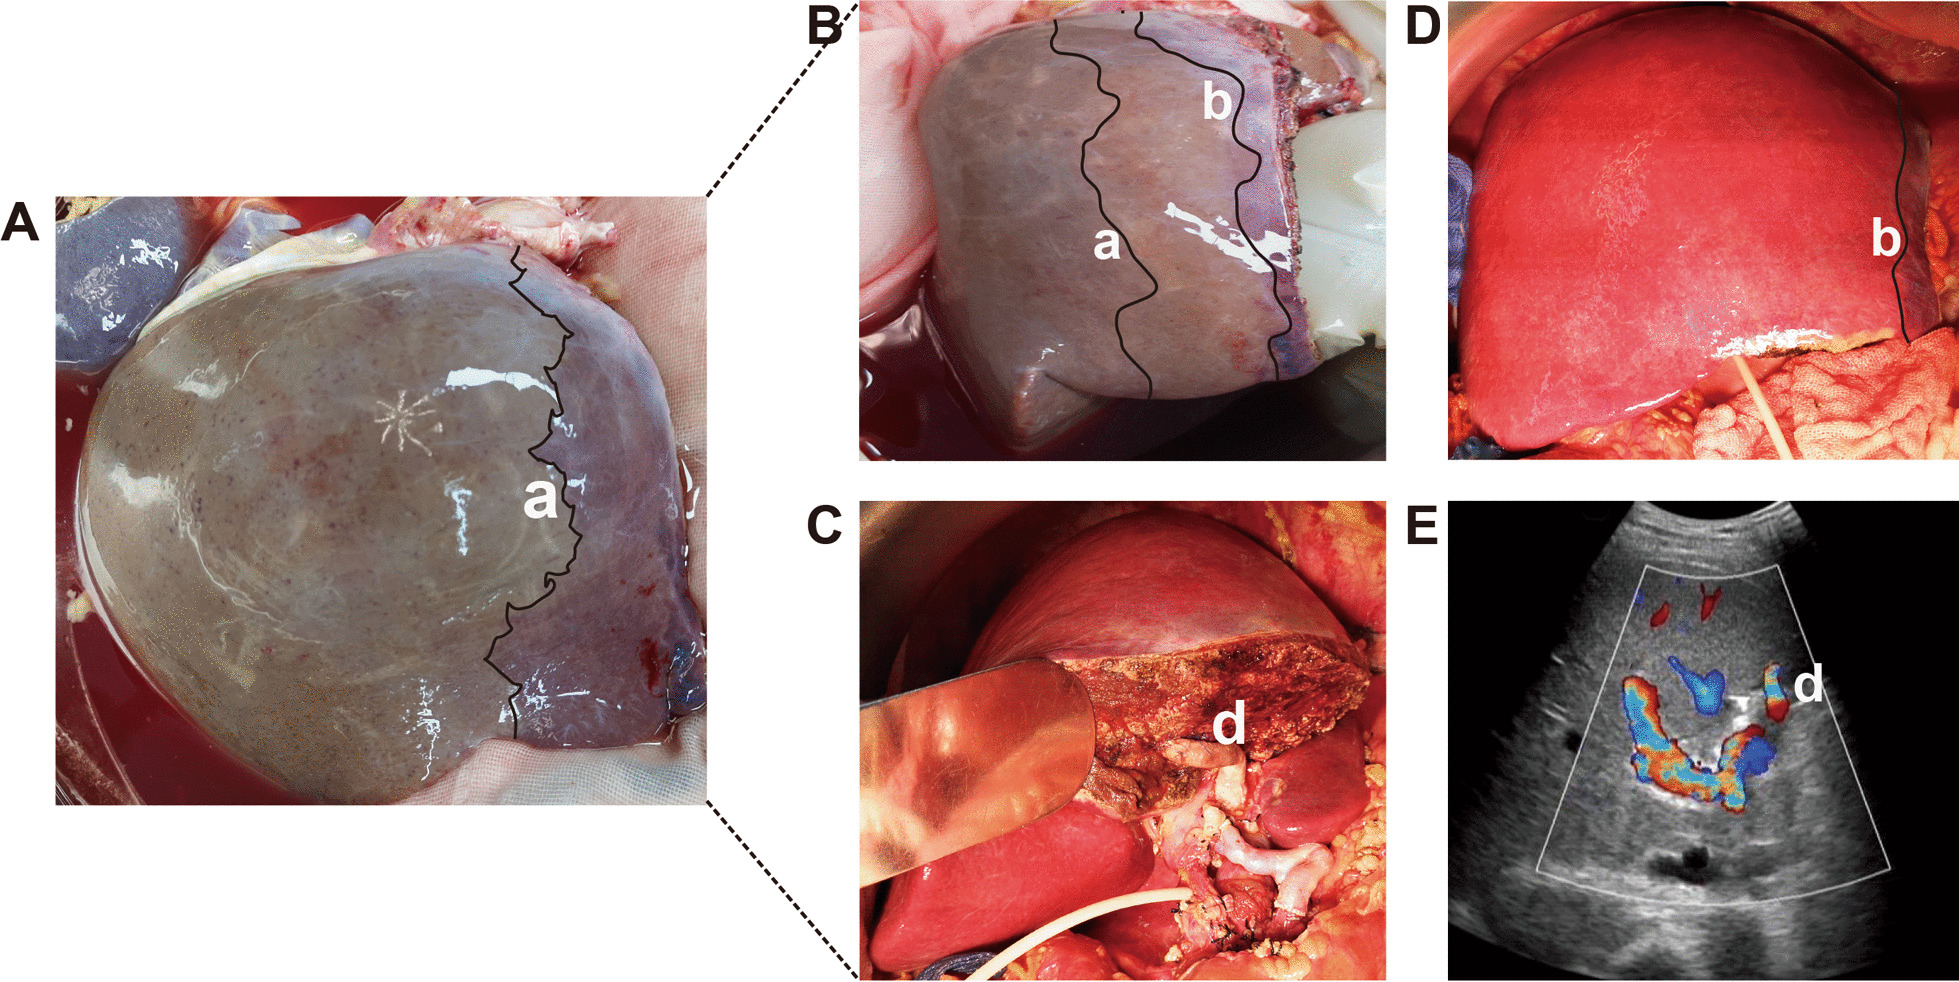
The ischemic of extended right grafts in SLT before and after IV-segment portal vein reconstruction. A The ischemic region in the ERG and ‘a’ mean the ischemic area. B The IV segment was reconstructed and we can find that the ischemic region was decreased. ‘b’ stands for the ischemic region. C The reconstruction vein in the extended right graft, ‘d’ means the reconstruction vein. E The reconstruction vein was tested by ultrasound, we can see the blood vessel patency (d)
